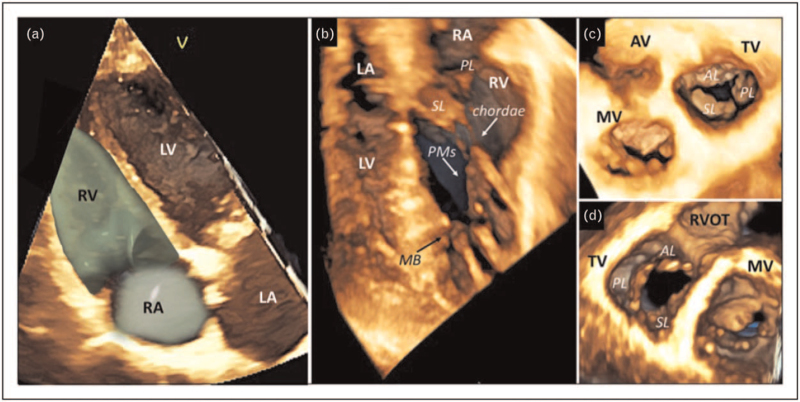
Three-dimensional assessment of the right ventricle and right atrium using transthoracic echocardiography (a). Evaluation of the subvalvular apparatus using transthoracic three-dimensional dataset showing tricuspid valve leaflets, chordae, papillary muscles and the moderator band (b). Three-dimensional echocardiographic display of the tricuspid valve form the atrial perspective (c) and ventricular perspective (d). AL, anterior leaflet; AV, aortic valve; LA, left atrium; LV, left ventricle; MB, moderator band; MV, mitral valve; PL, posterior leaflet; PMs, papillary muscles; RA, right atrium; RV, right ventricle; RVOT, right ventricular outflow tract; SL, septal leaflet; TV, tricuspid valve.
